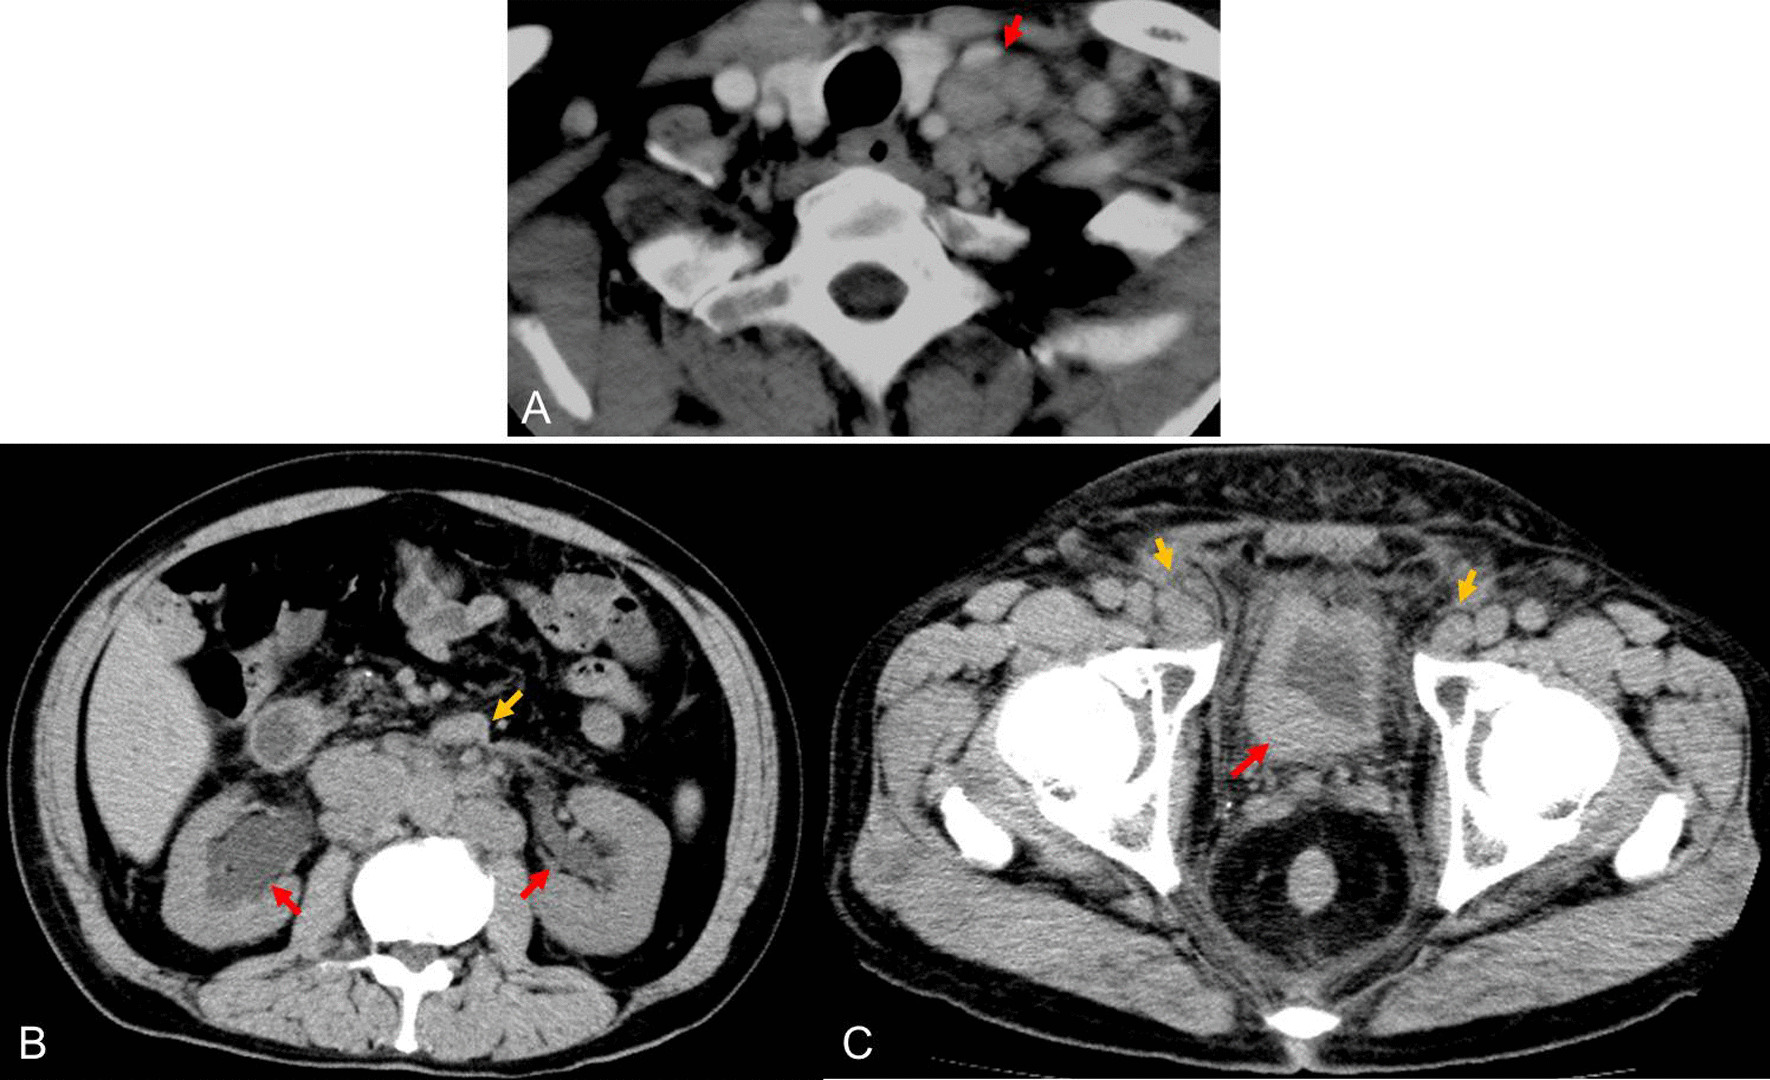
Enhanced computed tomography scans. A Enhanced computed tomography scan of left supraclavicular lymph node (red arrow). B Abdominal enhanced computed tomography scans. Bilateral hydronephrosis (red arrow) and paraaortic lymphadenopathy (yellow arrows) were observed. Pelvic enhanced computed tomography scans. C An ill-defined lesion in the bladder was observed (red arrow), and bilateral inguinal lymphadenopathy was observed (yellow arrow)
